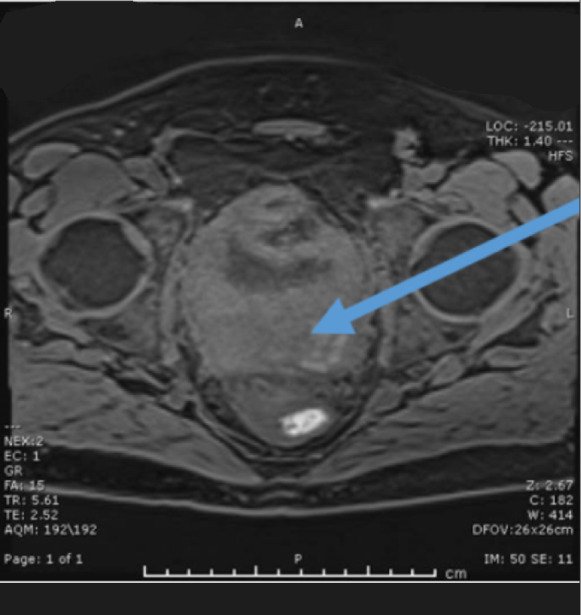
Cross-sectional magnetic resonance imaging scan showing marked posterior bladder wall thickening. Space in the upper left and right corners has been obscured for patient confidentiality purposes.
